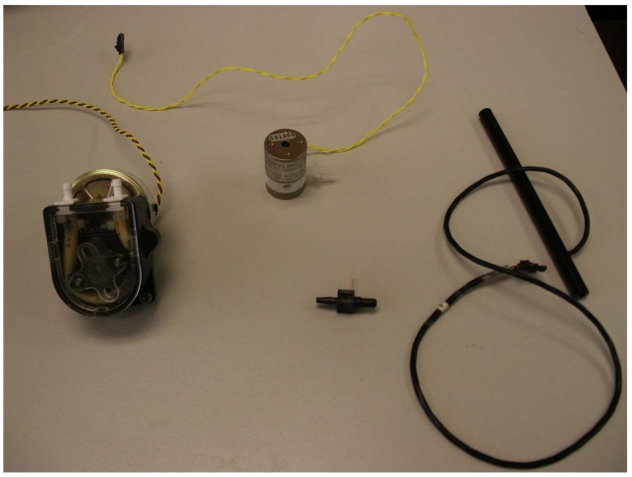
The major components of the fluid system – clockwise from left: peristaltic pump, solenoid valve, 545 CT cell (with custom connector installed), DP cell (before installation of custom circuit board).
